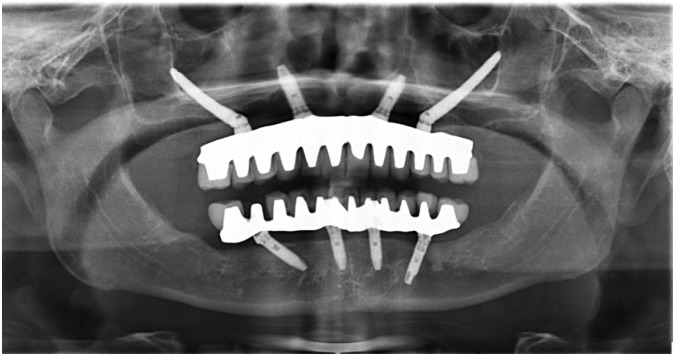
Panoramic radiograph of implants anchored in the zygomatic bone.
